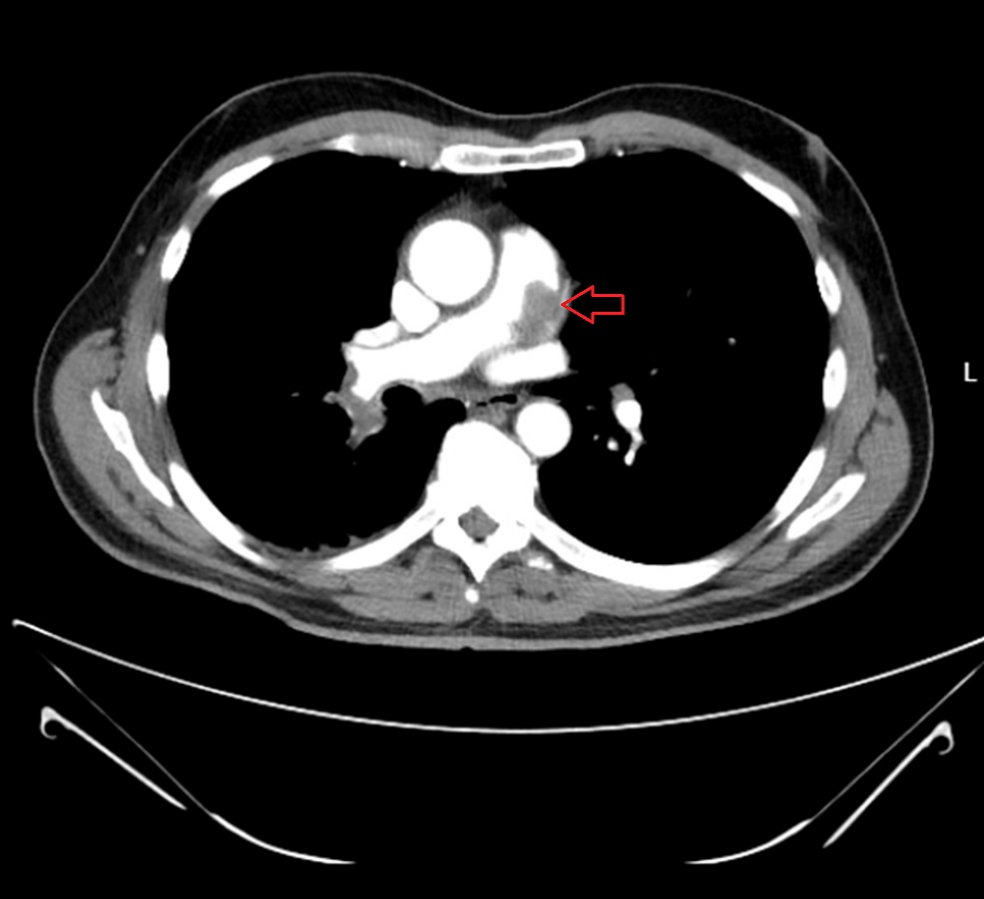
CT pulmonary embolus (PE) with filling defect within the main pulmonary artery (arrow) CT: computed tomography
